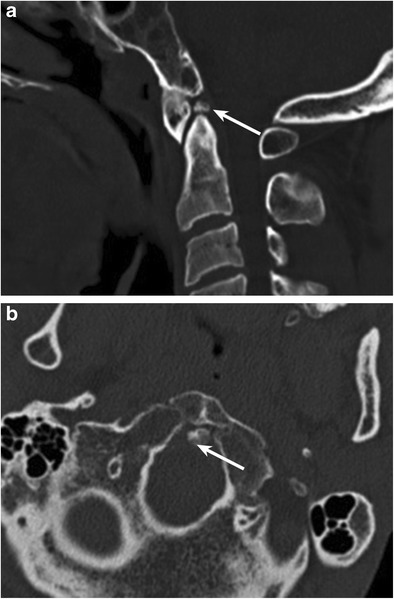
Persistent ossiculum terminale: Sagittal (*a*) and axial (*b*) images from a non-contrast CT of the cervical spine in a 32 year old demonstrate a small, well-corticated ossific density along the superior margin of the dens (*arrows* on images *a* and *b*). This likely represents the persistent ossiculum terminale, which arises as a result of failure of fusion of the secondary ossification centre with the superior aspect of the dens. This entity can be mistaken for a remote type I fracture of the dens
